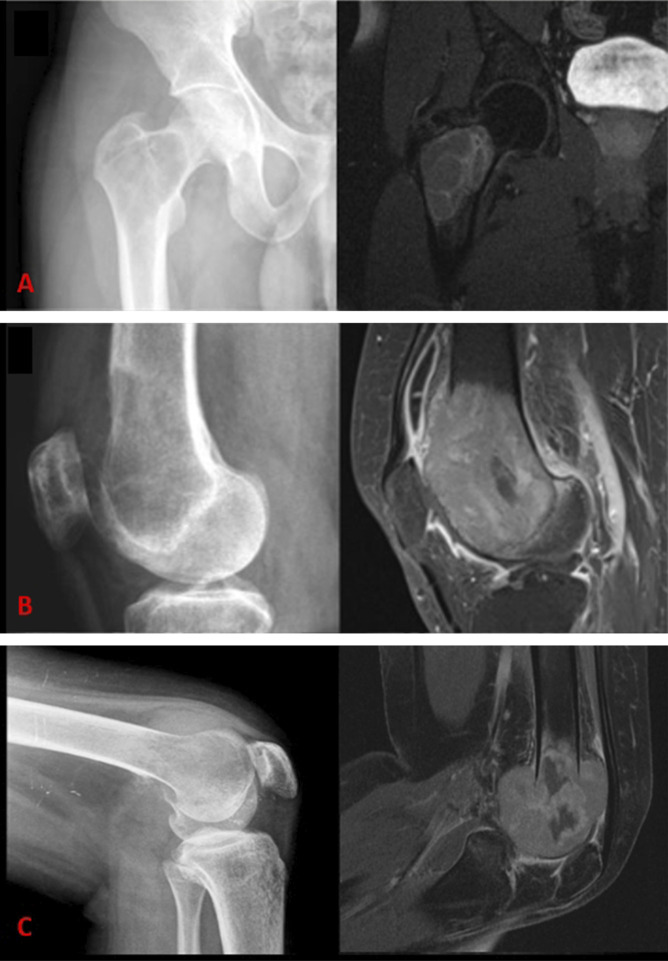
Representative examples are shown of novel classification system (A) grade 1, (B) grade 2, and (C) grade 3 GCTs of bone with paired radiographs and cross-sectional imaging.
